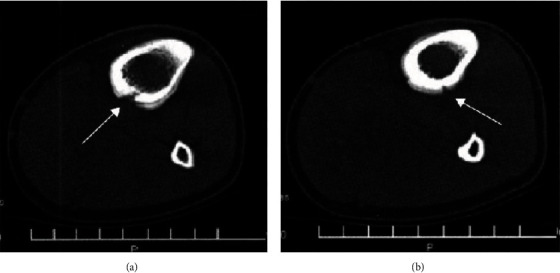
The patient's middle and upper tibial motion fracture image diagnosis based on CT cross-section slice and (b) CT cross-section slice.
